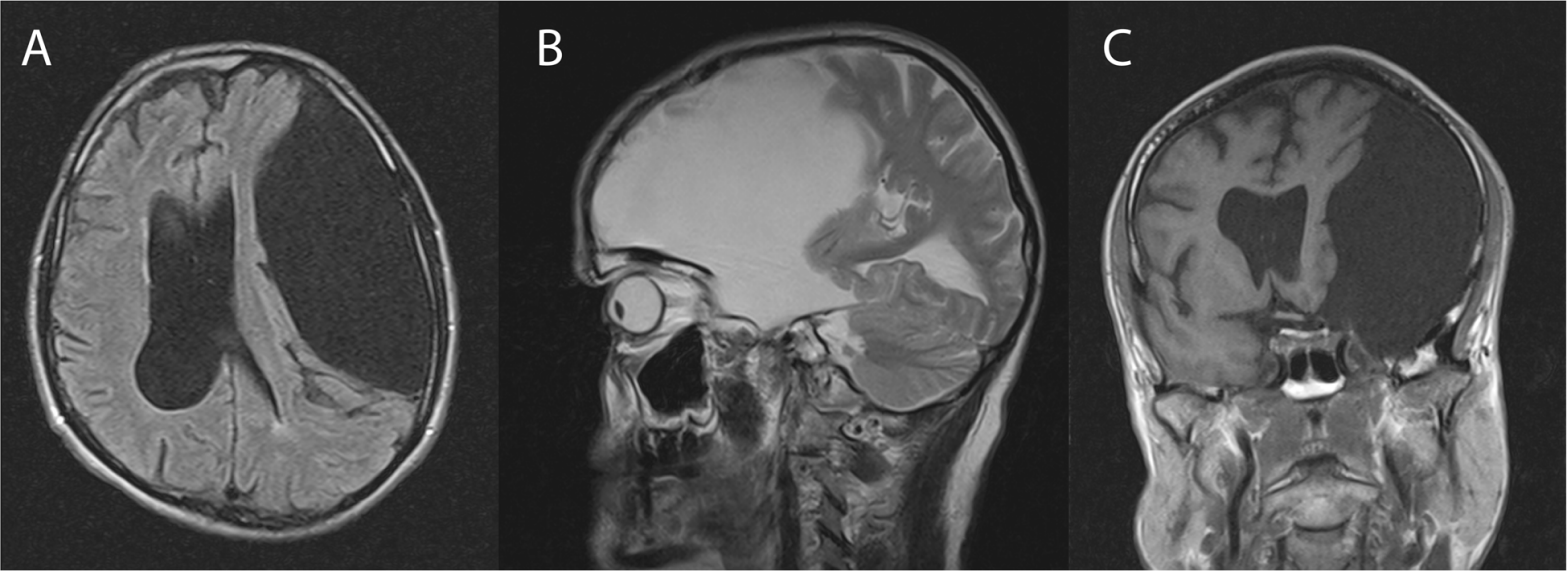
Brain MRI showing a large left frontotemporal cerebrospinal fluid-filled cyst with a cross-sectional area of 6 × 14 cm compatible with a Type III arachnoid cyst after the Galassi classification of middle cranial fossa arachnoid cysts: a FLAIR axial, b T2 TSE sagittal, and c T1 SE coronal view
